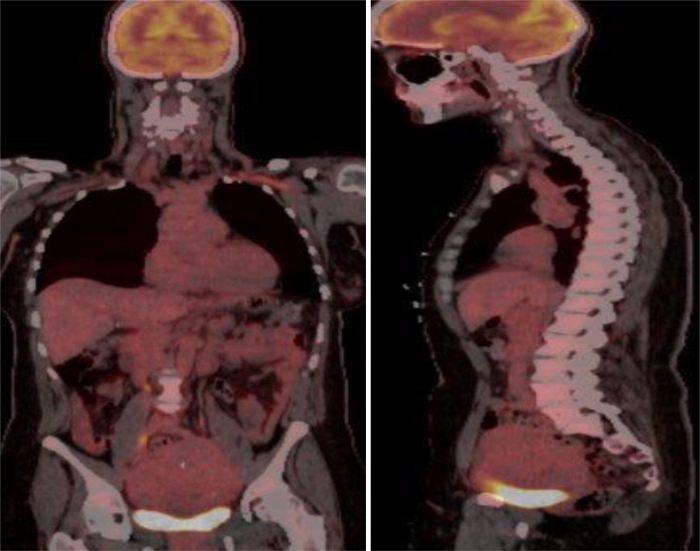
Whole-Body PET/CT Findings PET/CT demonstrating absence of fluorodeoxyglucose (FDG) uptake within the IVC and RA mass, supporting a benign process making a metabolically malignancy less likely. IVC = inferior vena cava; PET/CT = positron emission tomography/computed tomography; RA = right atrium.
